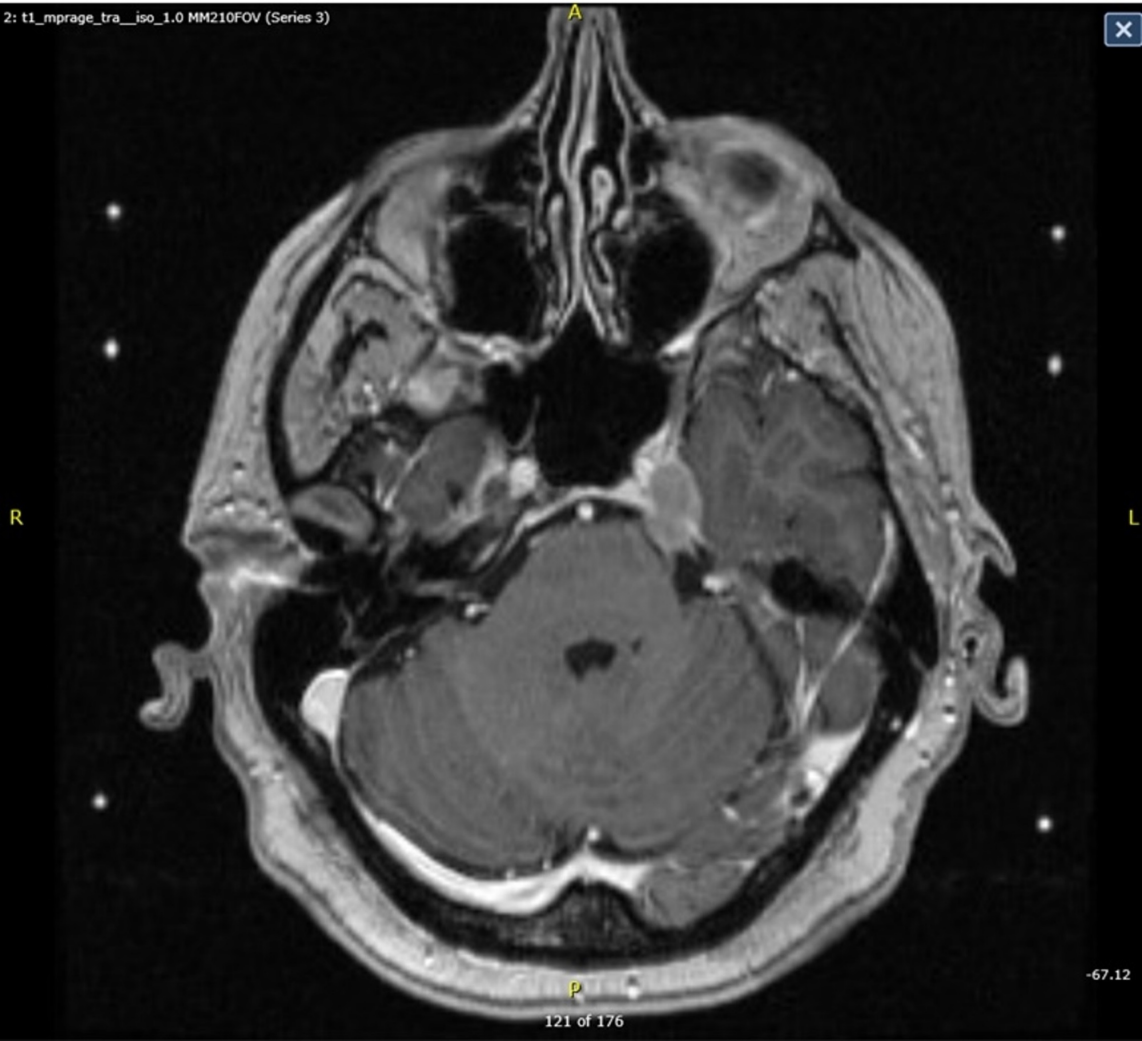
Pre-treatment Gamma Knife MRI MRI: magnetic resonance imaging
